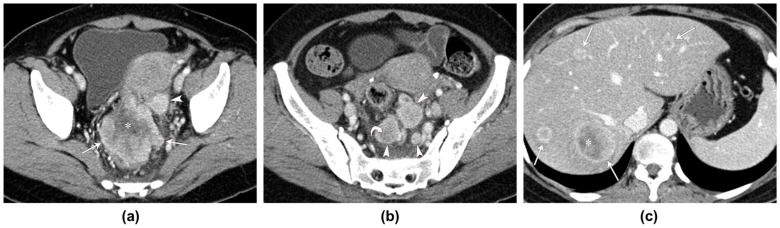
A 54-year-old woman with squamous cell carcinoma of the rectum. (a,b) Contrast-enhanced portal venous phase CT images demonstrate an infiltrative, heterogeneously well-enhanced bulky mass (arrows) in the upper rectum with central necrosis (asterisk) and invasion of the uterus. CT images also show multiple large metastatic lymph nodes (arrowheads) with some central necrosis (curved arrow). (c) The contrast-enhanced portal venous phase CT image depicts multiple peripheral well-enhanced metastatic nodules (arrows), with some central necrosis (asterisk) in both hepatic lobes.
